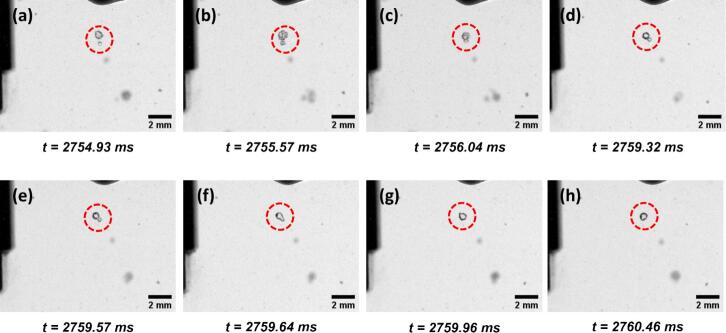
Bubble coalescence in pure DI water during UST. The red circle in each figure indicates the position of an example bubble of interest. Time values represent the elapsed time after the onset of ultrasonication. (For interpretation of the references to colour in this figure legend, the reader is referred to the web version of this article.)
